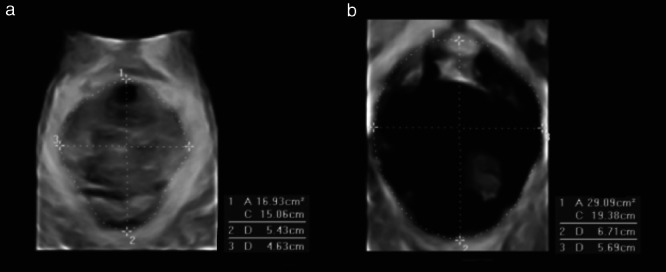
Ultrasound images of levator hiatus at rest (a) and on maximum Valsalva maneuver (b), on which area, anteroposterior diameter and coronal diameter were measured.
